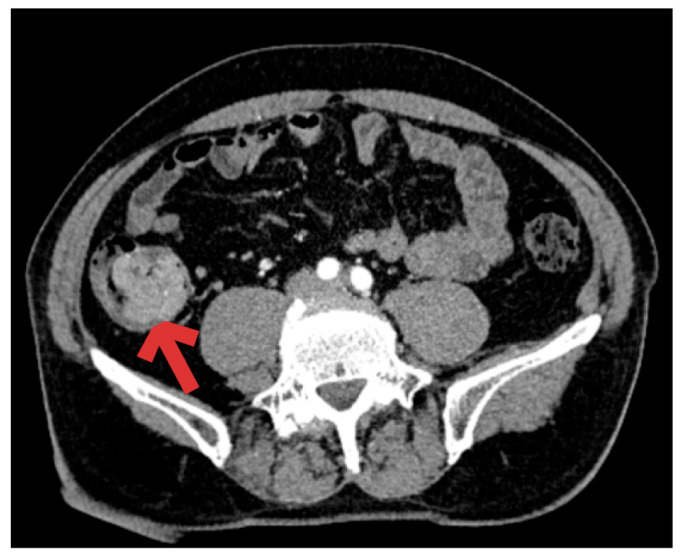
CT image—arrow indicates the cecal tumor.
